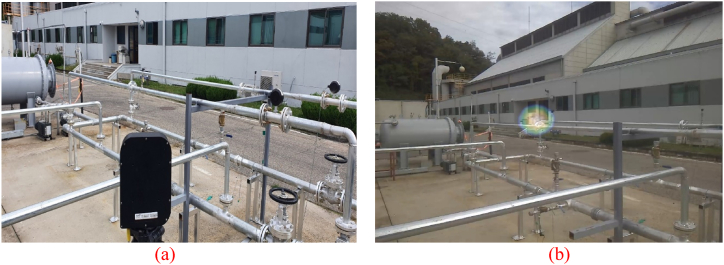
(a) Experiment set-up for case #1. (b) The ultrasonic camera detects the leaky flange without noise.
